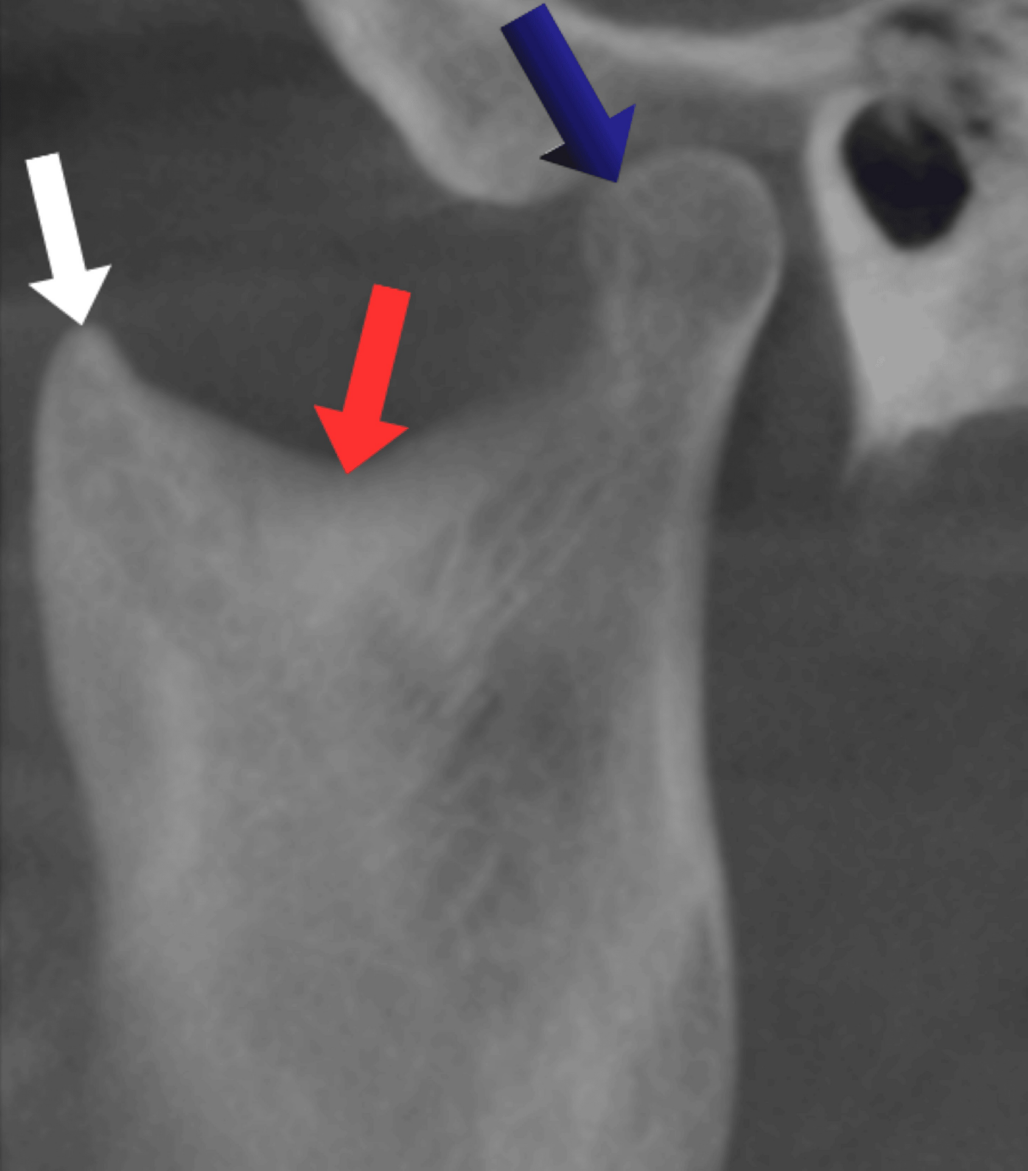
Morphology of coronoid (white arrow), condyle (blue arrow), and mandibular notch (red arrow) as assessed in sagittal view of CBCT. This figure depicts cone-beam computed tomographic image of a patient included in this study and used with the patients' permission. CBCT, cone-beam computed tomography
